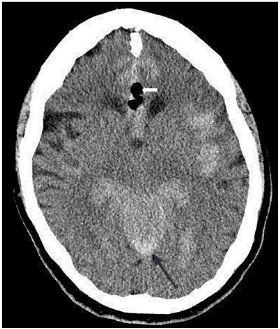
Computed tomography scan of the head showing pneumocephalus (white arrow), and subarachnoid hemorrhage (black arrow).
